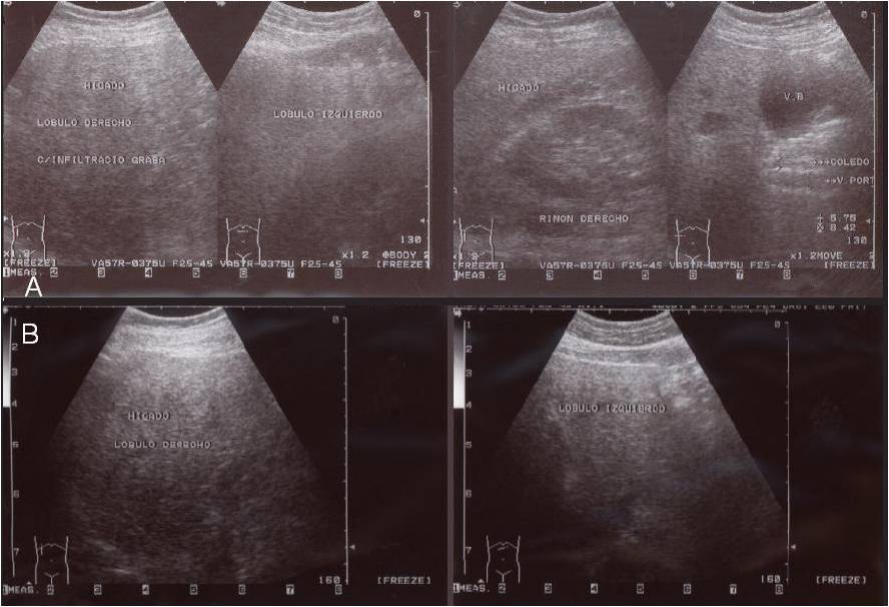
(A) Patient with non-alcoholic fatty liver disease detected by abdominal ultrasonography. An increased hepatic parenchymal echotexture by fat infiltration could be appreciated ("brilliant liver"). (B) The same patient after treatment with Spirulina maxima. Notice the attenuation of the previous sonographic pattern.
